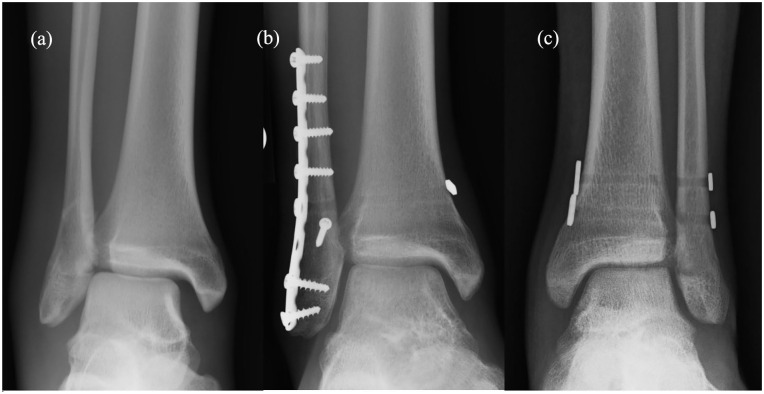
(a) Plain radiograph of Weber B type distal fibular fracture and associated syndesmotic disruption, (b) immediate postoperative radiograph of same patient treated with fibular fixation and suture button syndesmotic fixation, and (c) immediate postoperative radiograph of another patient who sustained isolated syndesmotic injury treated with two-suture button fixation constructs.
